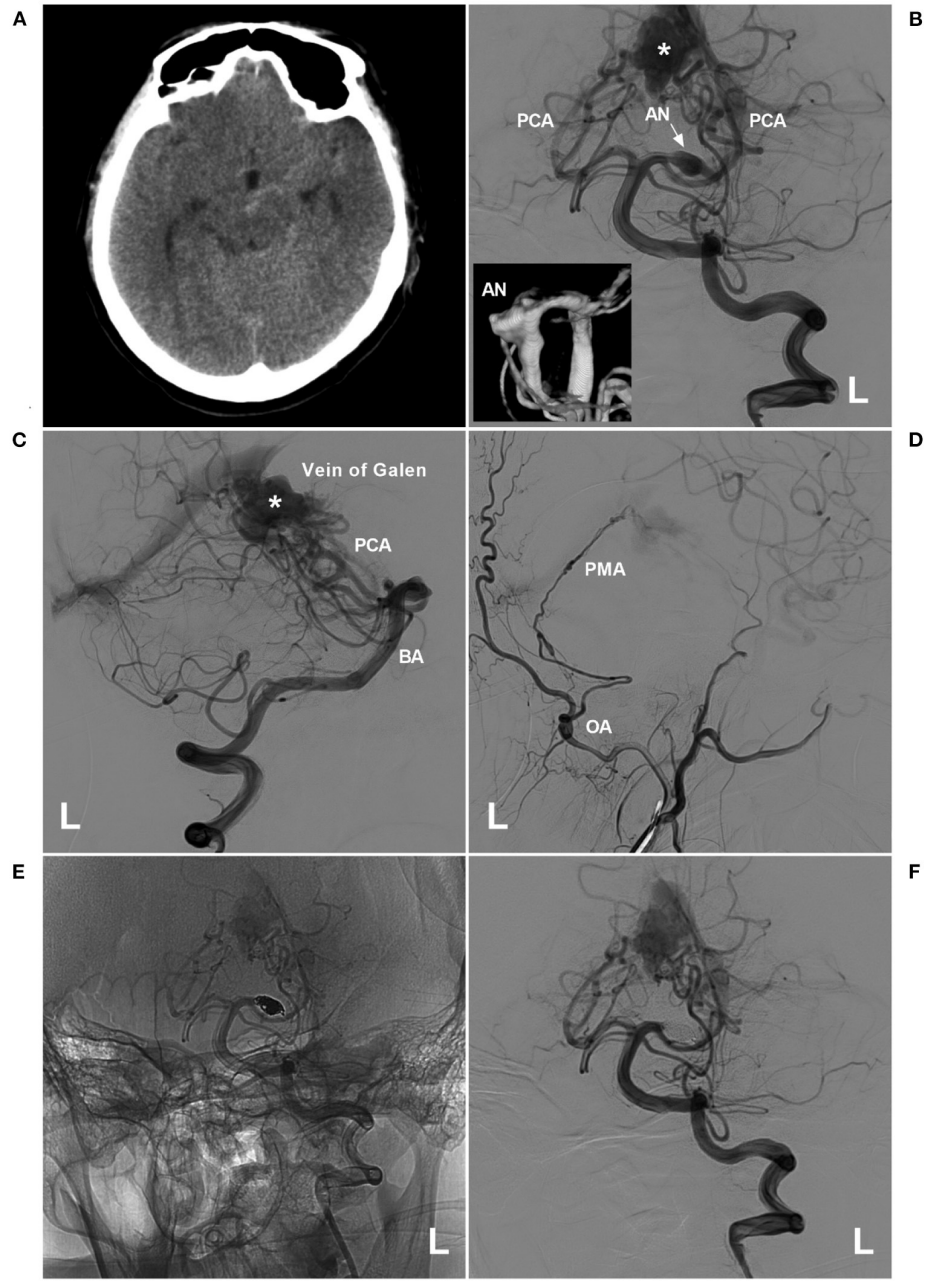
PAO for a proximal PCA aneurysm in the vein of Galen aneurysmal malformation. (A) Computed tomography showing a subarachnoid hemorrhage beside the left midbrain. (B,C) Angiograms of the left VA (B was the anteroposterior view, C was the lateral view) showing a choroidal type of vein of a Galen aneurysmal malformation, and the asterisk indicated a dilated vein of Galen; in B, an aneurysm (arrow and AN) located at the proximal PCA as the feeding artery to the vein of Galen aneurysmal malformation. In the picture inset, the aneurysm was irregular. (D) Angiogram of the left external carotid artery showing that the PMA from the OA also acts as the feeding artery. (E,F) Unsubtracted (E) and subtracted (F) angiograms of the left VA showing that the aneurysm was occluded by coiling PAO. AN, aneurysm; BA, basilar artery; L, left; OA, occipital artery; PAO, parent artery occlusion; PCA, posterior cerebellar artery; PMA, posterior meningeal artery; VA, vertebral artery.
